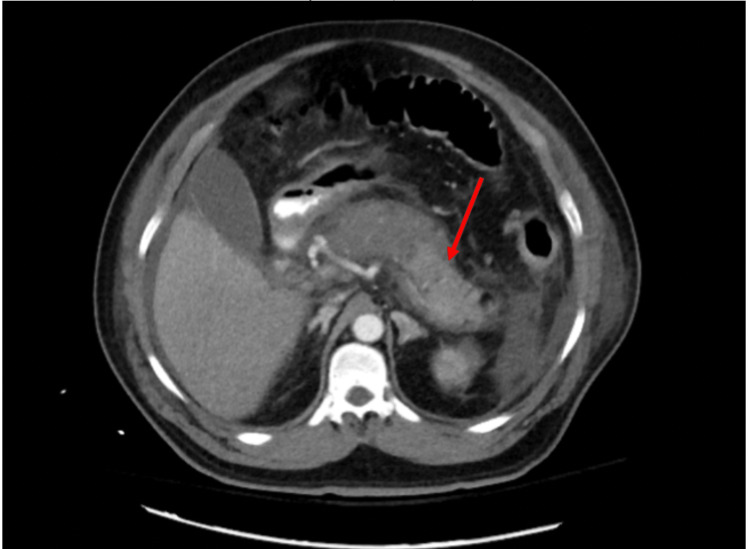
Computed tomography scan of the abdomen with contrast on day three showing edematous pancreas with areas of necrosis around the head, body, and tail (red arrow).
